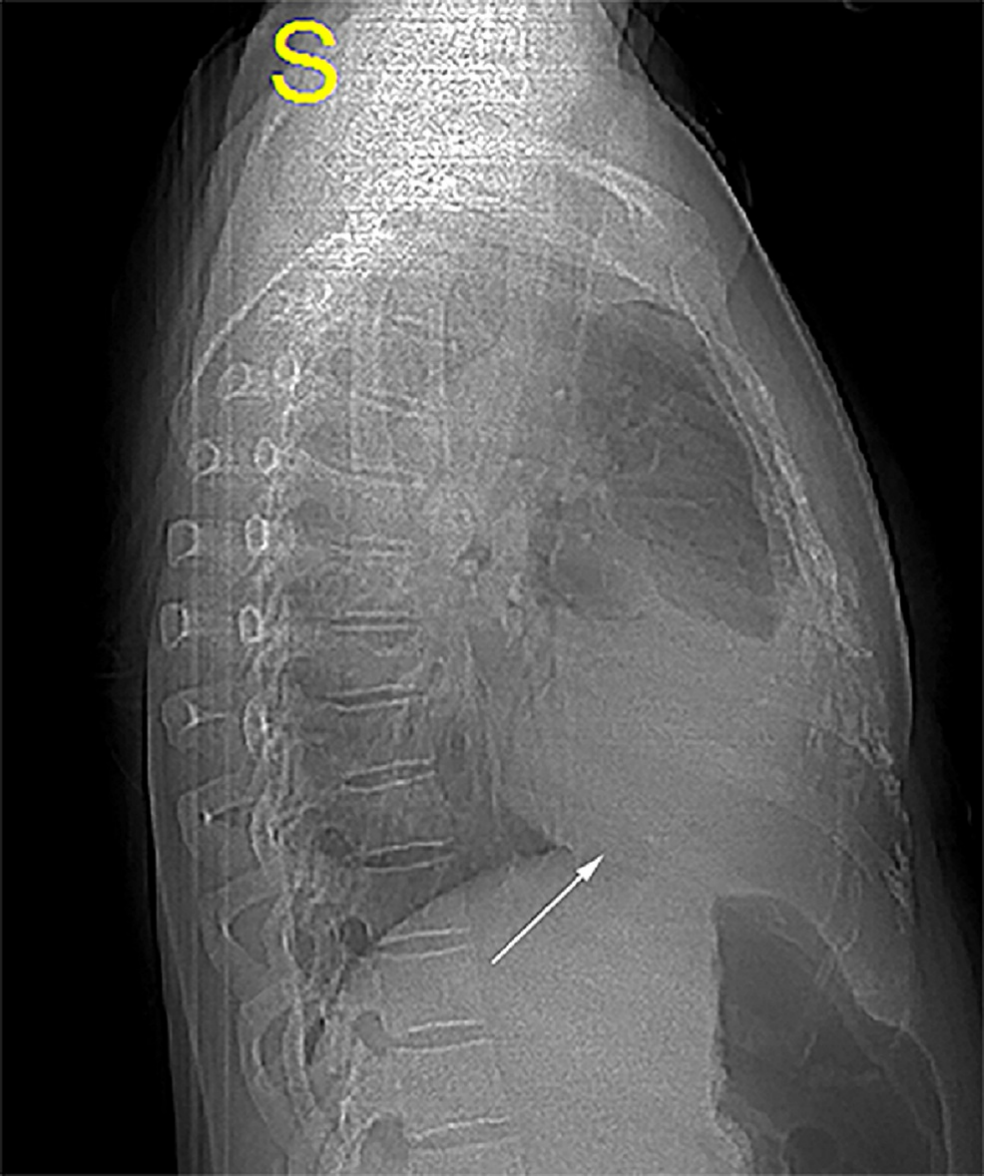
Chest X-ray (right lateral view) showing the extent of the right pleural effusion
